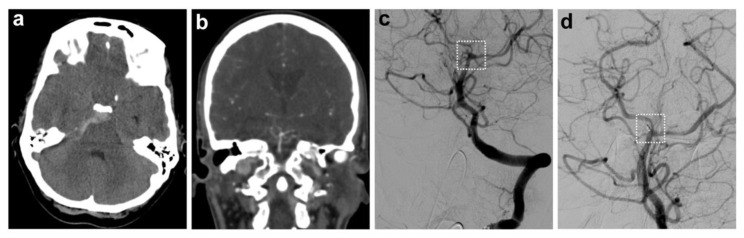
Exemplary case of a patient with a perimesencephalic bleeding pattern and an aneurysmal pathology. (a) Non-contrast CT depicting a perimesencephalic pattern of subarachnoid hemorrhage with predominantly right-sided distribution. (b) Subsequent CT angiography with evidence of a tiny saccular aneurysm of the SCA on the right, which was initially thought to be a prominent basilar artery. (c) DSA confirmed the aneurysm of the right SCA; (d) shows the coiled aneurysm (marked with a white dashed box).
